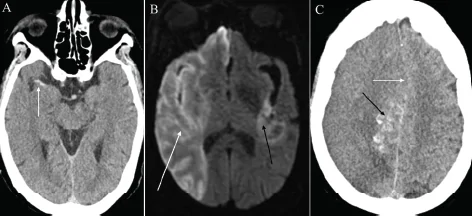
Axial views of the patient’s brain imaging over a period of 24 hours. (A) Initial computed tomography (CT) of the head without contrast revealed a hyperdense right middle cerebral artery (arrow). (B) Diffusion-weighted magnetic resonance imaging of the brain showed acute infarct in nearly the entire right middle and anterior cerebral artery territories (white arrow), as well as acute infarct of the left insular cortex and temporal lobe of the left middle cerebral artery territory (black arrow). (C) Subsequent CT head without contrast showed hemorrhagic transformation with intraparenchymal hemorrhage (black arrow) and 7 mm of midline shift (white arrow).
